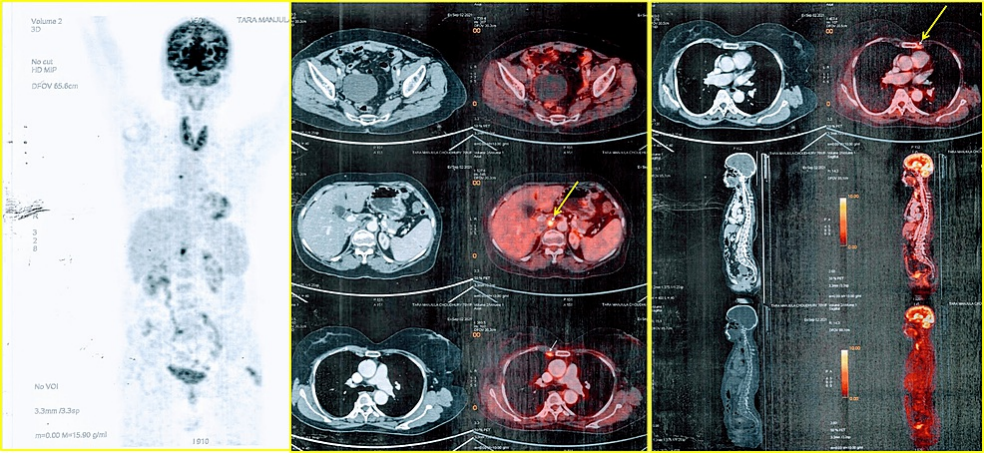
The PET/CT shows a platinum-sensitive relapse of ovarian carcinoma (the arrow indicates sites of abnormal uptake) PET/CT: Positron emission tomography-computed tomography
